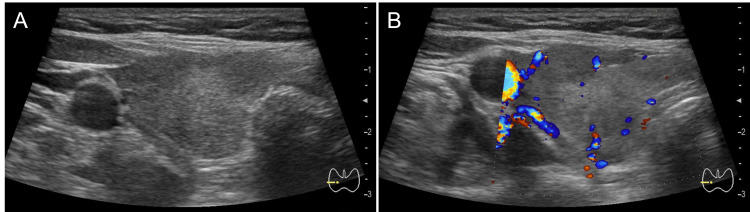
An ultrasound of right thyroid lobe. (A) Ultrasound of the right thyroid lobe showing a heterogeneous thyroid gland with a hypoechoic area. (B) Ultrasound of the right thyroid lobe showing decreased Doppler flow.
